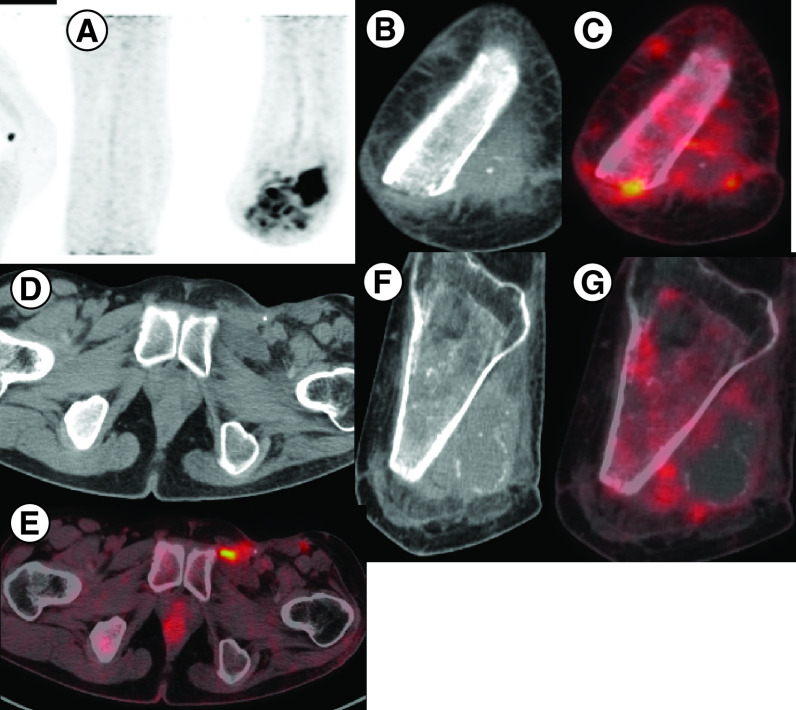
PET Scan imaging showing radiographic findings after treatment with tazemetostat. (A) MIP image showing focal increased tracer uptake in the left inguinal region and in the left knee joint region. (B & C) Previously seen cutaneous nodules in baseline image show resolution in size and metabolic activity in the present study. (D & E) Ulcerated subcentimetric left inguinal lymph nodes on CT showing increased FDG uptake on fused PET-CT scan. (F & G) Sclerosis in left tibia showing decreased FDG uptake compared with previous scan with overall findings suggest partial resolution of the disease following therapy. CT: Computed tomography; MIP: Maximum intensity projection.
